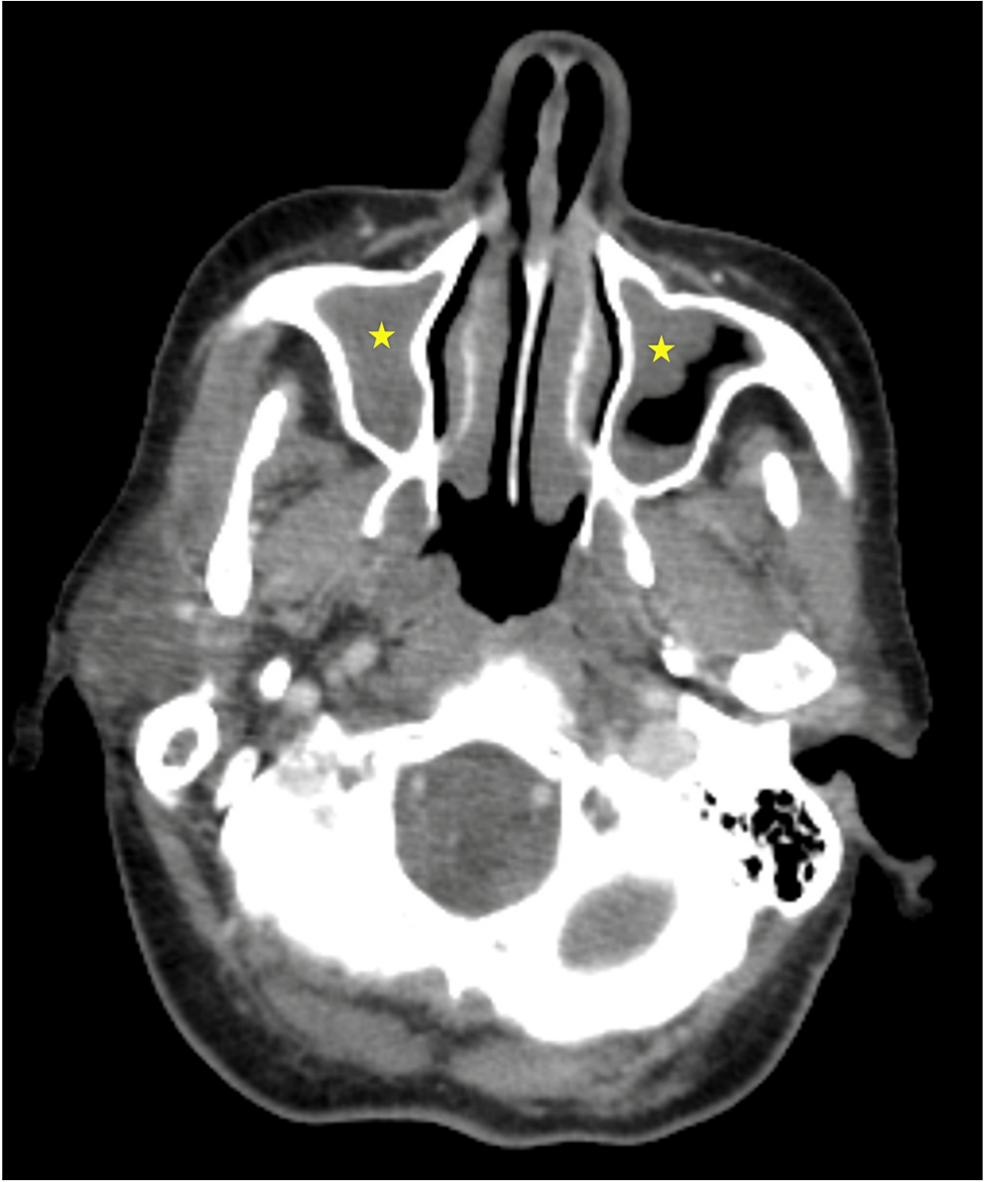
Maxillary computed tomography (CT) scan showing acute inflammatory changes in the maxillary sinus (stars).
